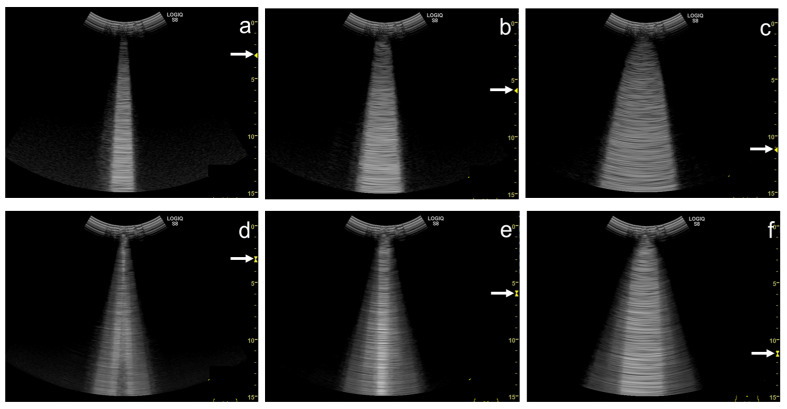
Ultrasound images obtained with the curvilinear transducer. The vertical artifact becomes wider as the focal point (arrow) is moved to deeper levels (a–c). When spatial compound imaging is enabled, three lines appear to overlap each other (a,d). The three lines become wider as the focal point (arrow) is moved to deeper levels (d–f).
